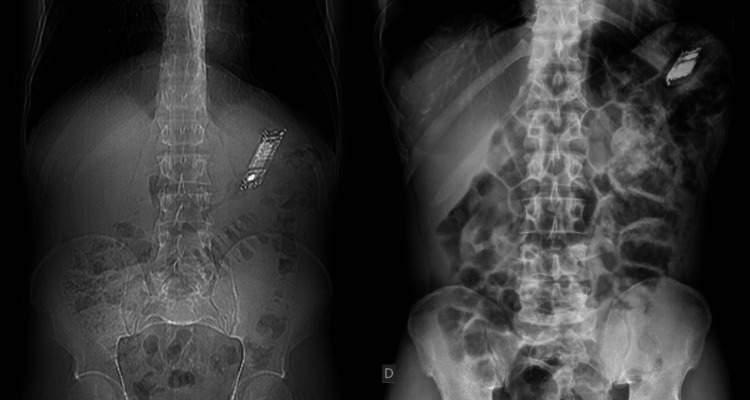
Radiographs showing the cell phones in the patients’ gastrointestinal tracts as obtained in the abdominal radiograph. In all these patients, the cell phones were clearly visible.
